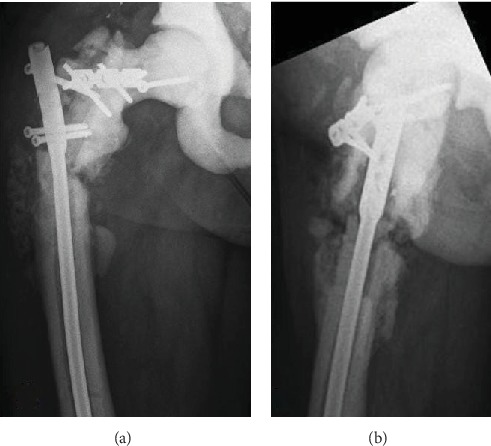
Anteroposterior (a) and lateral (b) radiographs showing the pull out of the intramedullary nail of the femur.
